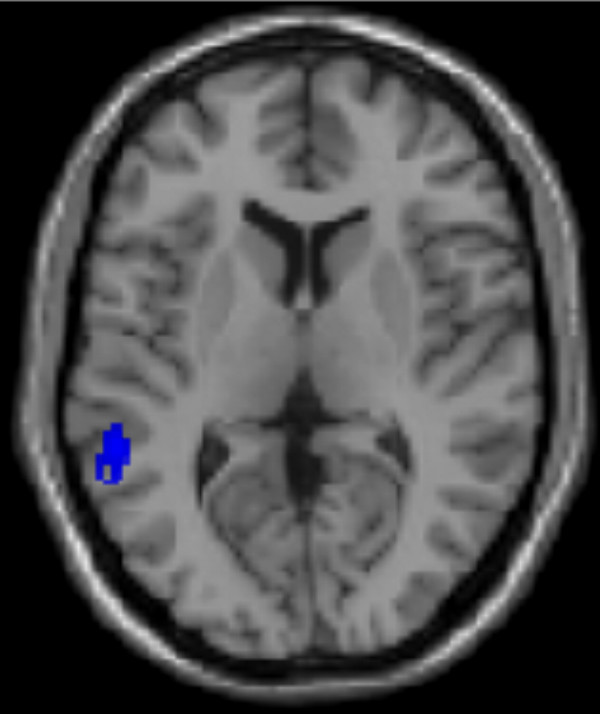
Involvement of the left superior temporal gyrus in PC27 superimposed on the canonical single-subject MR image of SPM2 in an axial plane (blue, negative loading).
